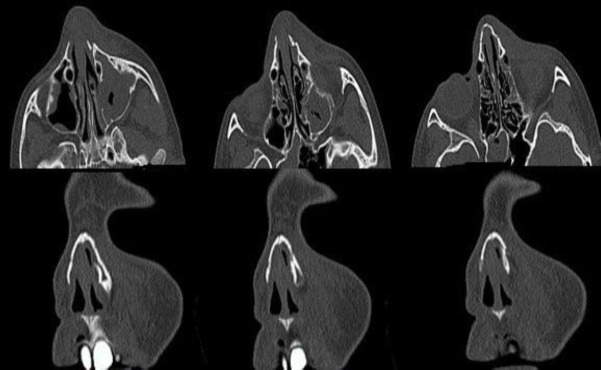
Preoperative maxillofacial CT image of the patient
